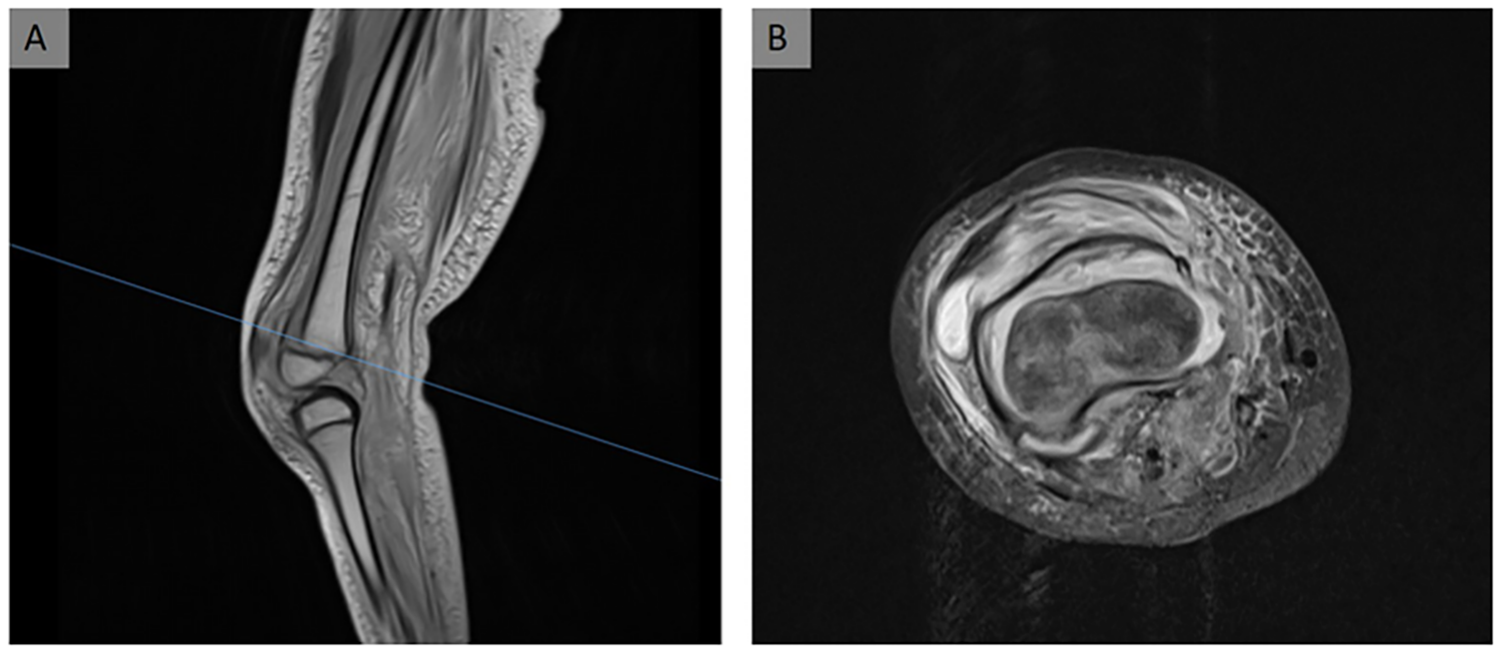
MRI scan of the patient's right knee. (A) Epiphyseolysis of the distal femur in the sagittal view. Because the epiphyseal scar is not crossed and the fracture line is along the epiphyseal scar, this is classified as a Salter–Harris type 1 fracture. (B) Axial view of the right knee. The femoral condyle appears to be dislocated ventromedially from the femoral metaphysis.
